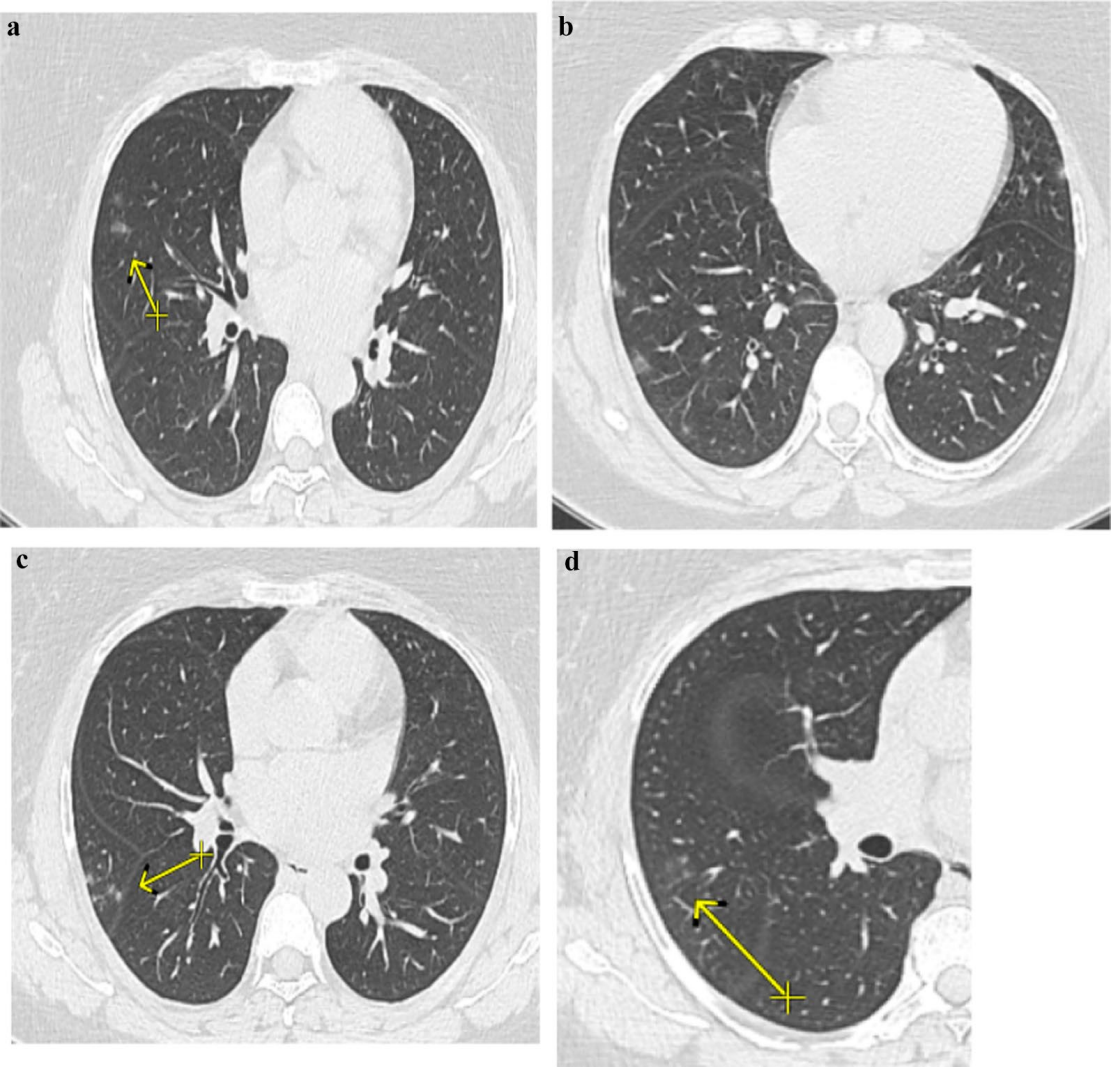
A 33-year-old male presented with anosmia, loss of taste, fever, diarrhea and myalgia at July 2020 and positive PCR for COVID 19 infection, a and b noncontrast CT axial cuts shows bilateral faint ground glass opacities. The patient diagnosed as COVID 19 infection with CT score severity: 6. Second infection dated June 2021 the patient presented with anosmia with positive PCR test for COVID 19 infection. c and d noncontrast CT chest images show right lower lobar patchy subpleural faint ground glass opacities. The patient diagnosed as COVID 19 re-infection with CT severity score: 2
